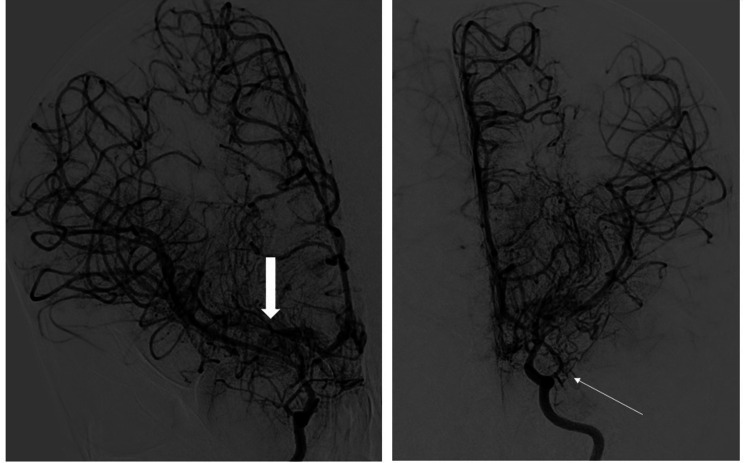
Conventional angiography demonstrates bilateral internal carotid arteries stenosis seen at the carotid terminus with multiple collateral formation compatible with a history of moyamoya disease (left). There is evidence of right middle cerebral artery M2 segment thrombosis compatible with MRI finding of acute infarction. There is evidence of left distal middle cerebral artery branches thrombosis (right).
